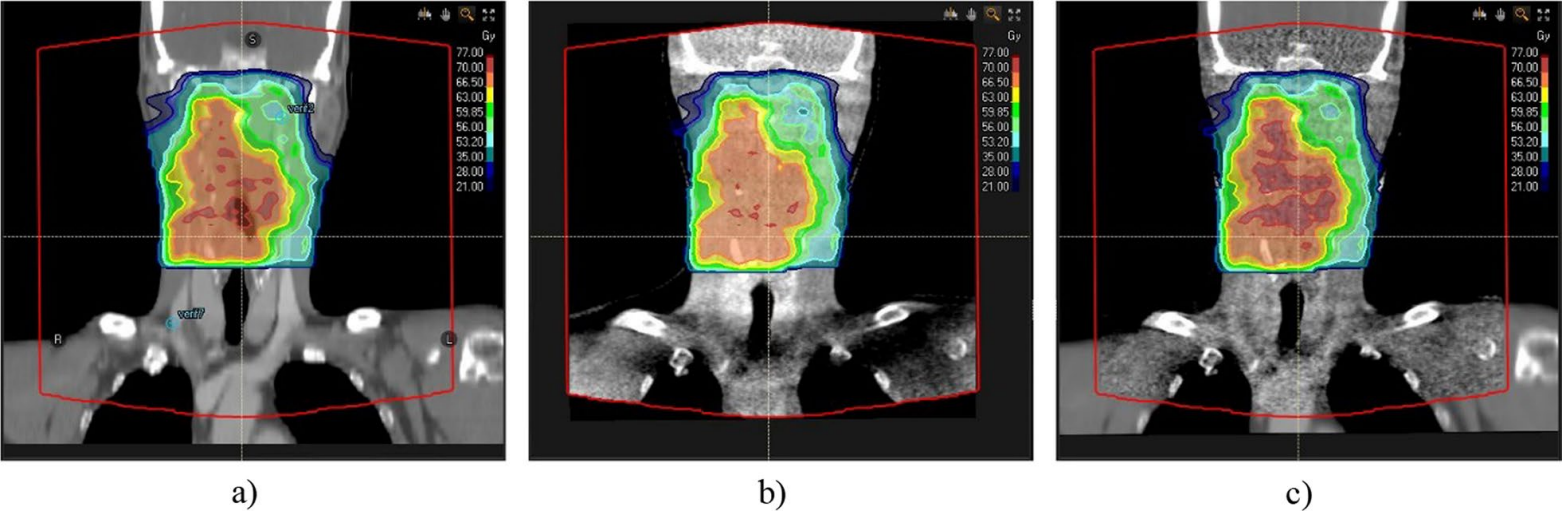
Screenshots of a HN case. The pCT is shown in a), the CBCT_b_ is shown in b) and the CBCT_c_ with the pCT stitched to the regions outside the FOV is shown in c). The FOV of the CBCT is annotated in red
